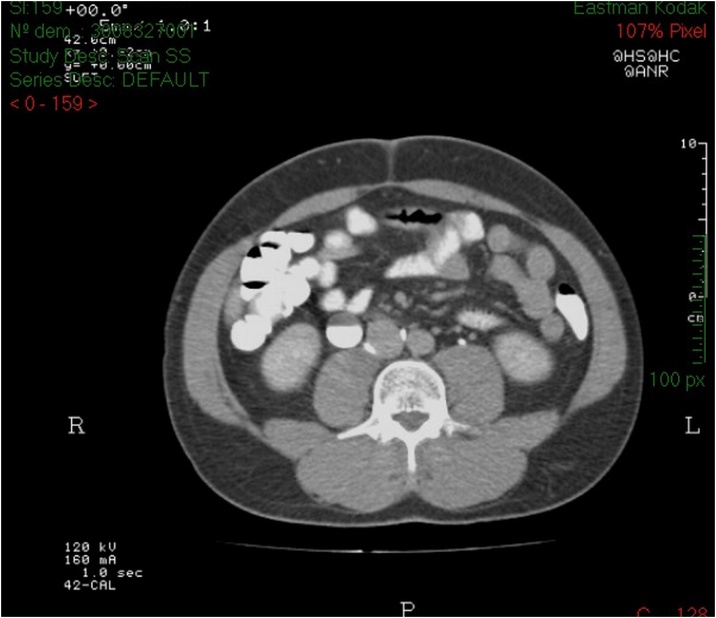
Computed tomography scan of patient 2 showing the presence of a retrocaval ureter.
